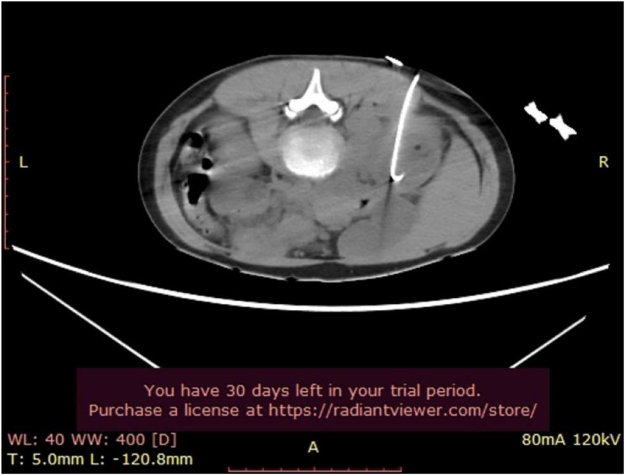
CT-guided drainage of the abscess: nephrostomy tube seen.
